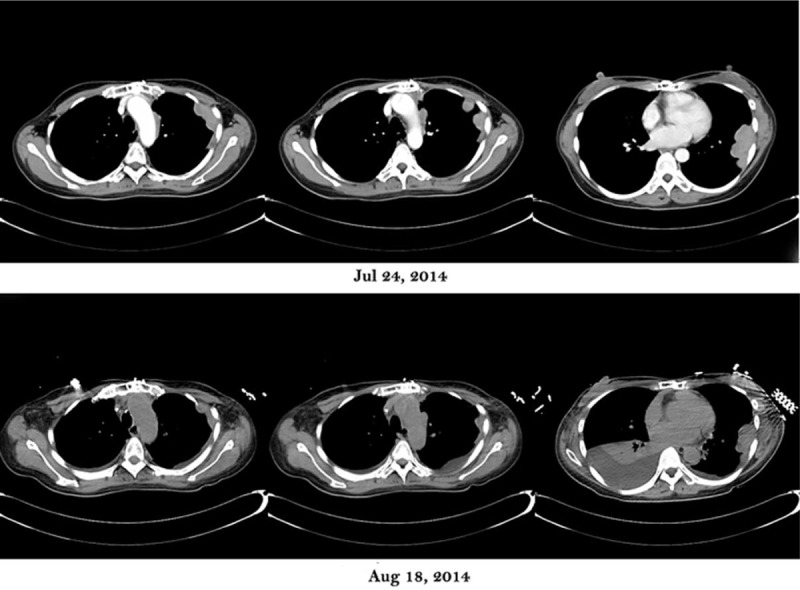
Comparison of CT scan between July 24, 2014, and August 18, 2014. A significant response of tumor following 3 sessions of plasmapheresis was indicated from CT scan. CT = computed tomography.
